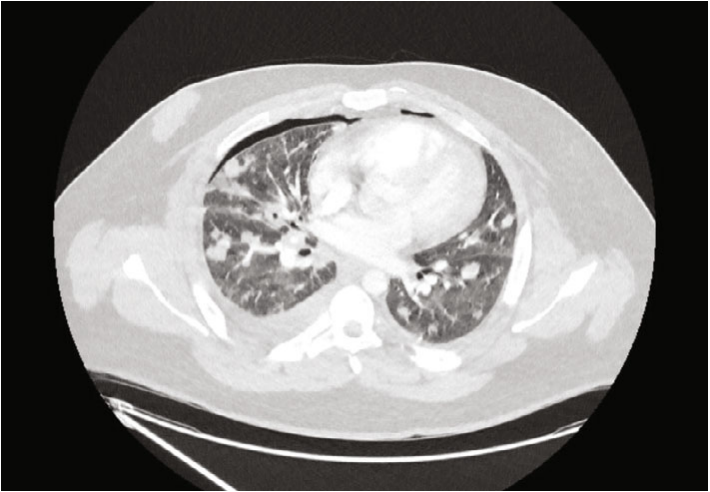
Transverse computed tomography thorax at admission (Day 1) showing right pyopneumothorax, left small pyopneumothorax, bilateral interlobar septal thickening and bilateral pulmonary nodules of varying size, some cavitating.
